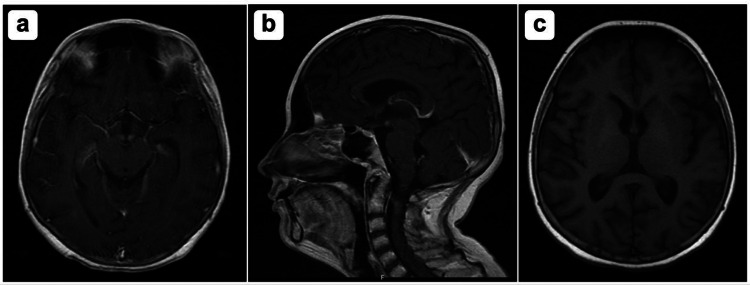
MRI with and without contrast showing: A) bilateral mild swelling and T2 hyperintense edema of the mammillary bodies. Additional symmetrical T2 hyperintense signal is noted along the dorsal medulla, extending to the Ponto medullary junction. B) There is also T2-flair hyperintense signal in the periaqueductal region and mild bilateral periventricular T2 hyperintensity. There is a small area of T2 hyperintense signal abnormality in the cerebellar vermis. C) Corresponding enhancement is noted, mainly in the bilateral mammillary bodies and mild enhancement along the bilateral dorsal medulla. Findings are highly suggestive of Wernicke encephalopathy/thiamine deficiency.
